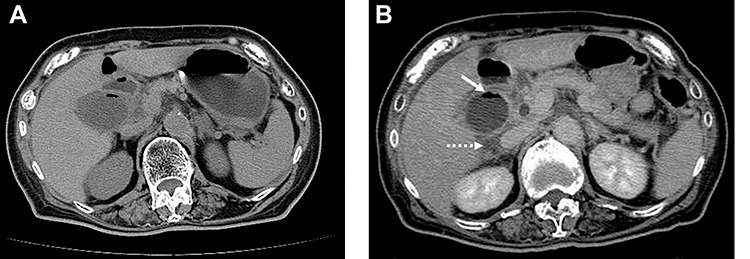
On admission, abdominal computed tomography (CT) scan showed a gas-fluid level in the gallbladder and surrounding fatty infiltration with ascites (A). On hospital day 2, abdominal CT revealed increased gas within the gallbladder (B, white arrow) and the development of pericholecystic fluid (B, dot arrow).
